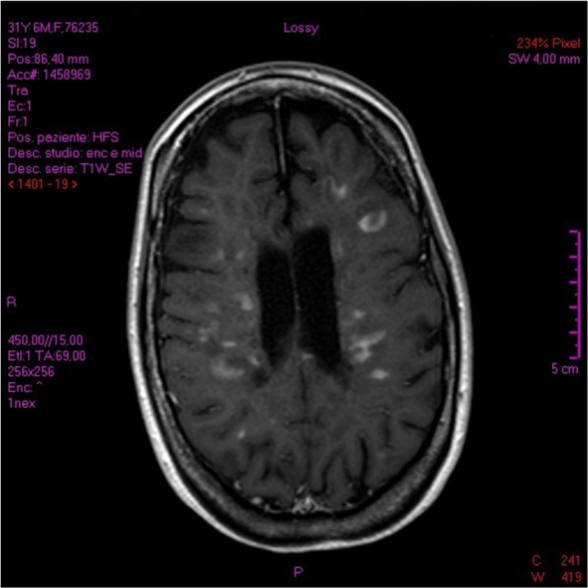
The pre-treatment brain MRI check-up demonstrated CELs. The main locations are supra- and infratentorial white matter, hemispheric white matter, cortical-subcortical junction, and periventricular white matter bilaterally
